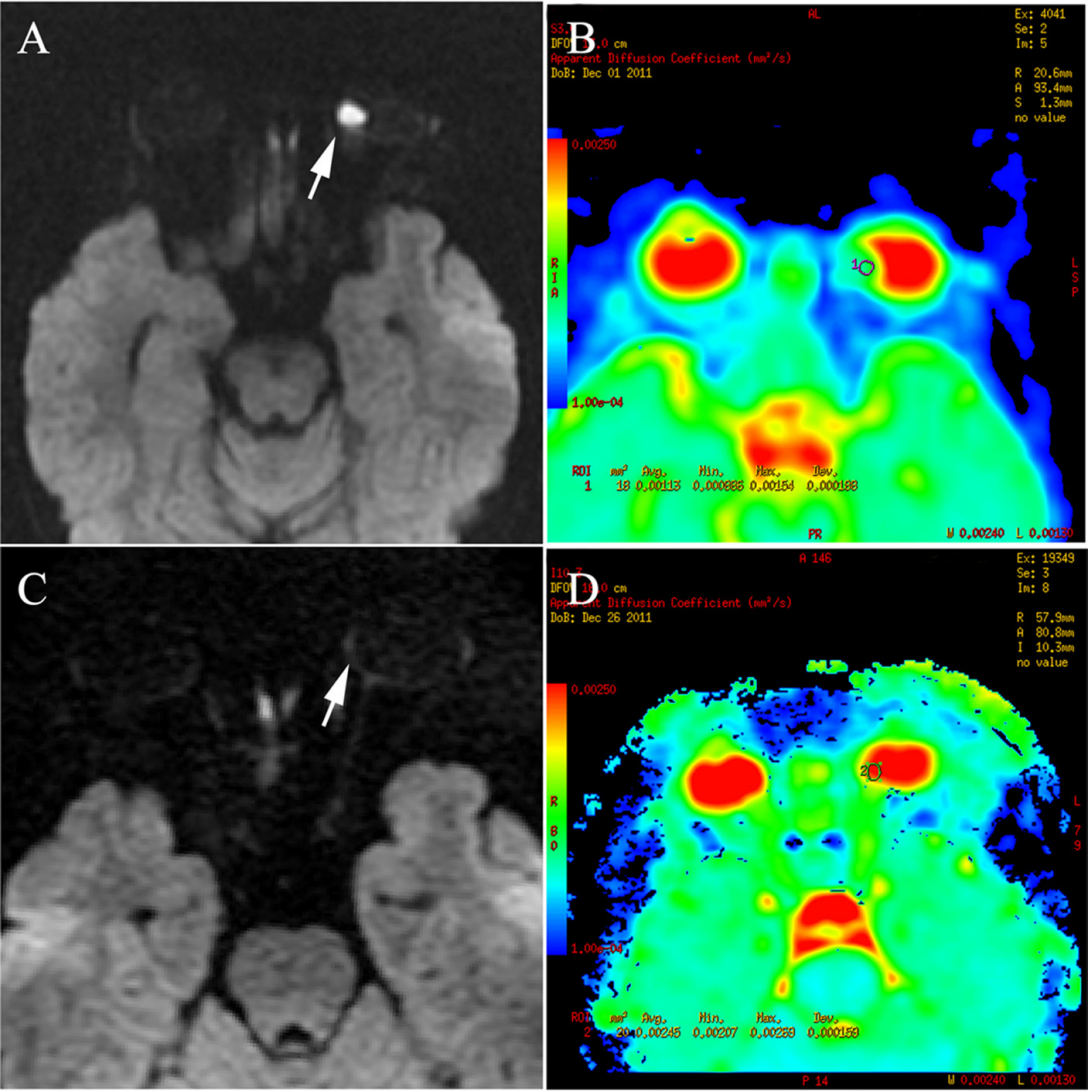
Retinoblastoma of the right eyeball in a 44-month-old female (patient 2) The DWI (A) shows hyperintensity in the tumor (arrow), indicating hyper-cellularity. The apparent diffusion coefficient (ADC) of this lesion was 1.13 × 10^−3^ mm^2^/s (B). After three cycles of intra-arterial chemotherapy (IAC), DWI shows hypointensity in the tumor region (C), with ADC of 2.45 × 10^−3^ mm^2^/s (D).
